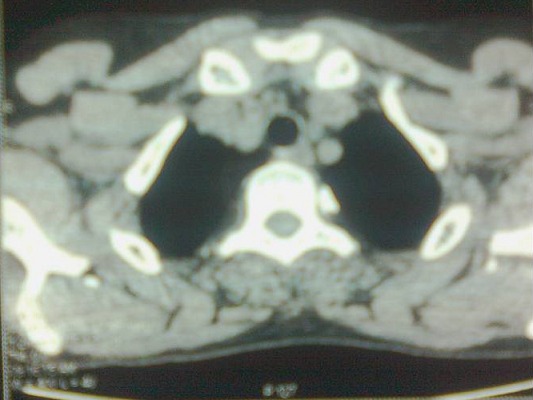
Mediastinal lymph nodes in Barety space and bilateral hilar lymphadenopathy on chest CT
